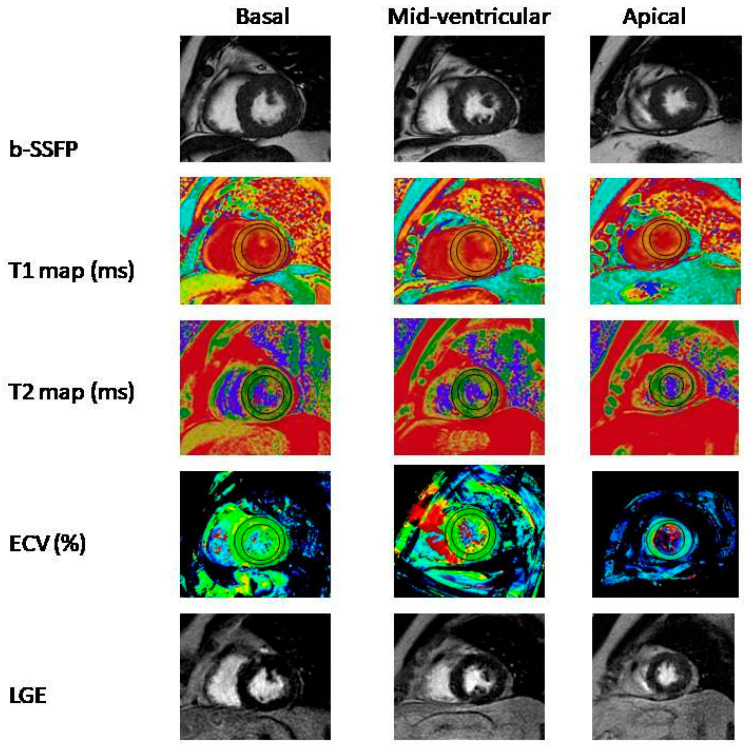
Cardiac MRI examination of a 35-year-old male patient diagnosed with COVID-19. The same slices of short axis view on basal, mid-ventricular, and apical segment (left-to-right) for b-SSFP (b-SSFP, balanced steady-state free precession), T1 mapping, T2 mapping, ECV (extracellular volume) map and LGE (late gadolinium enhancement) images (up-to-down). T1, T2, and ECV mapping values for entire segments were 1129 ± 8 ms, 41 ± 2 ms, and 30 ± 2%, respectively.
